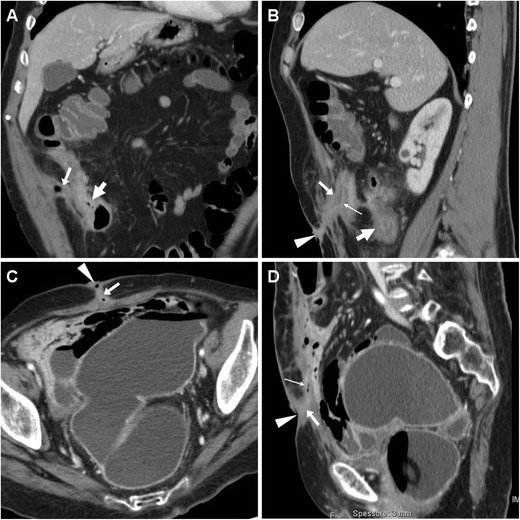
Two cases of spontaneous, low-output ECF in CD. In a 59-year-old man with a history of ileocecal resection nearly 20 years earlier, CT (A, B) identified a right-sided ECF as an enhancing “tram-track” fistula (arrows) with mucosal hyperenhancement (thin arrow), which departed from the thickened preanastomotic ileum (thick arrow) with recurrent CD and crossed the abdominal wall and subcutaneous fat to the EFO (arrowheads); repeated ileocolonic resection and ECF debridement were performed In a 62-year-old woman with several surgeries, water-enema CT (C, D - note distended rectosigmoid colon with rectal tube) showed a paramedian hypogastric ECF with mucosal hyperenhancement (thin arrow) and focally depressed EFO (arrowheads), communicating with the collapsed ileum; the patient did well on infliximab therapy
